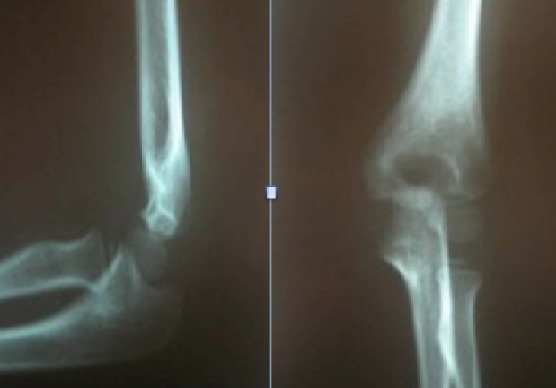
Radiographies chez le même enfant montrant une bonne consolidation osseuse
